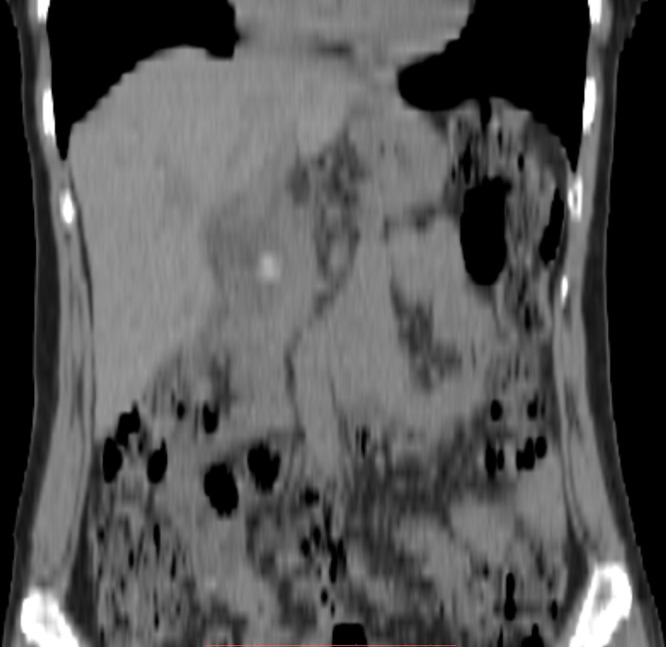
Non-contrast CT coronal reformatted image showing calcified cystic duct calculus.
